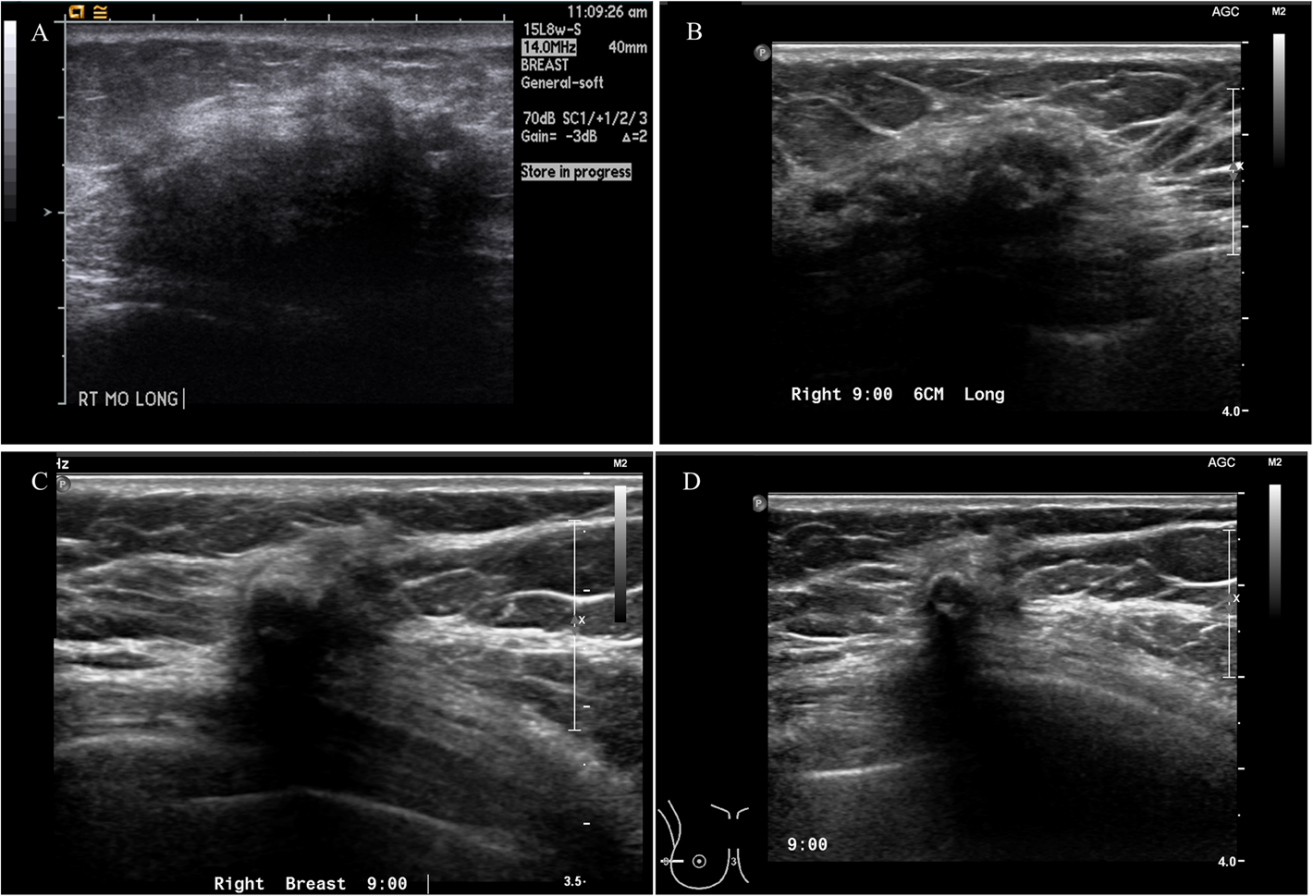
Consecutive changes in fat necrosis after breast reconstruction in ultrasonography. a Initially, fat necrosis was detected after 6 months from surgery as a mass of around 4 cm with an indistinct margin in the mid-outer portion of the right breast. Needle biopsy was performed, and fat necrosis was confirmed pathologically. b After 1 year from the detection of fat necrosis, the volume of the necrotic mass was decreased. And calcification appeared at the center of the fat necrosis area (arrowhead). c After 2 year from the occurrence of fat necrosis, the mass was shrunk further. d Although the mass became much smaller over time, calcification (arrowhead) remained at the fat necrosis area
